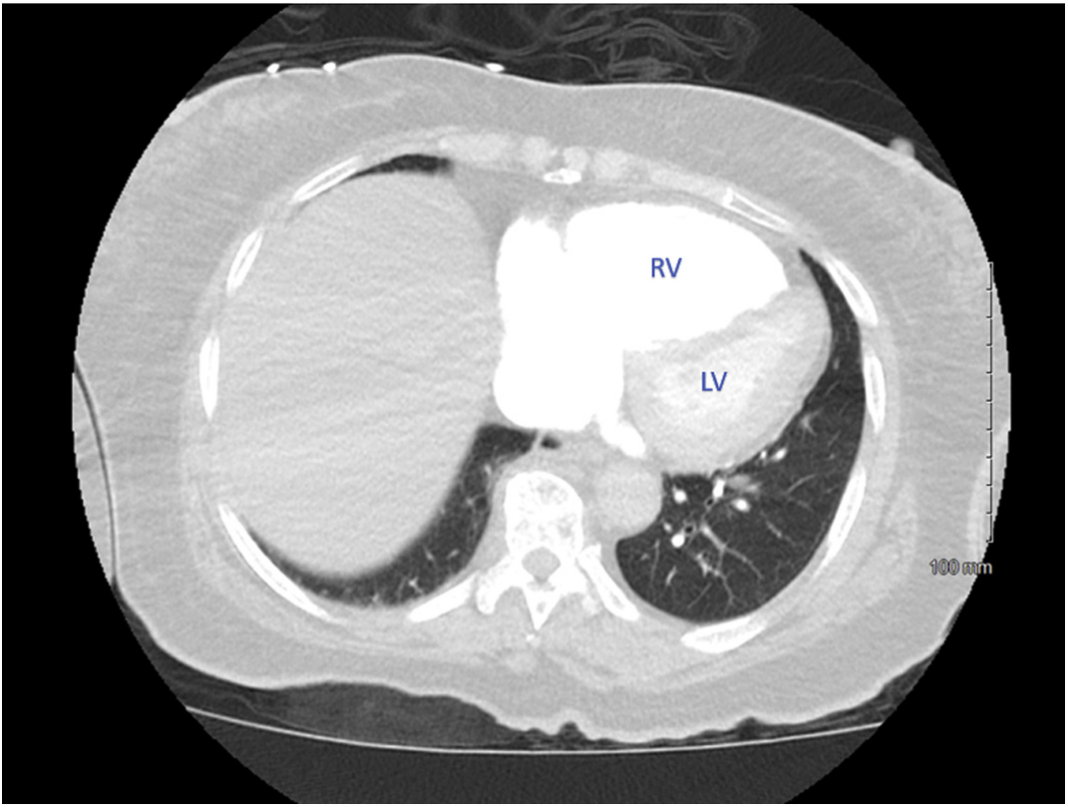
Dilation of Right Ventricle Dilated right ventricle (RV) on computed tomography scan. RV:left ventricle (LV) >1, consistent with RV strain.
